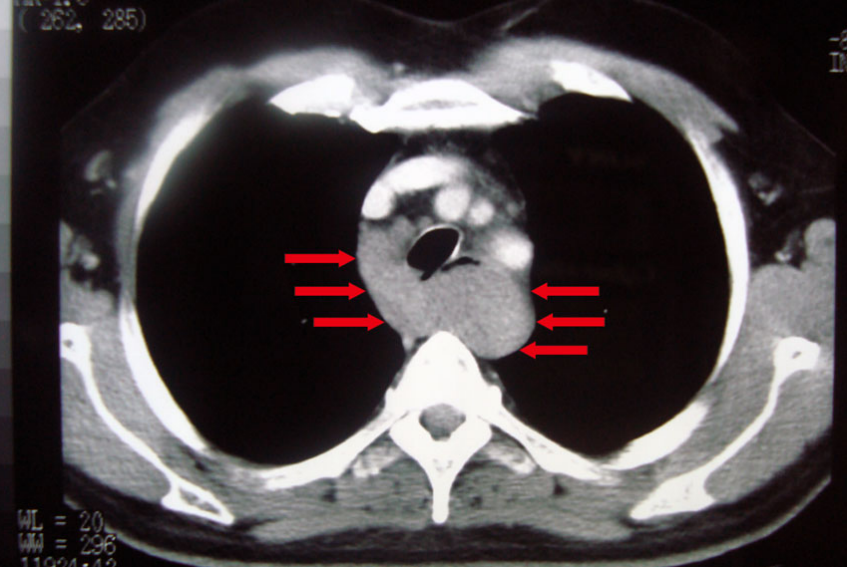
Computed Tomography of the chest revealing a large mass originating from median esophagus (red arrows).
